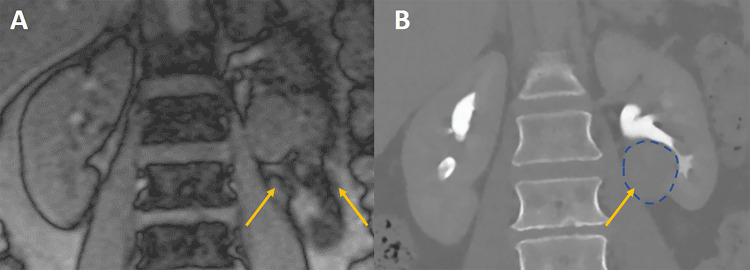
Coronal images of the thoracolumbar spine, capturing the kidneys A: magnetic resonance image (MRI); B: computerized tomography (CT) image. Arrows show a mass in the left kidney (outlined with a discontinuous superimposed circle).
